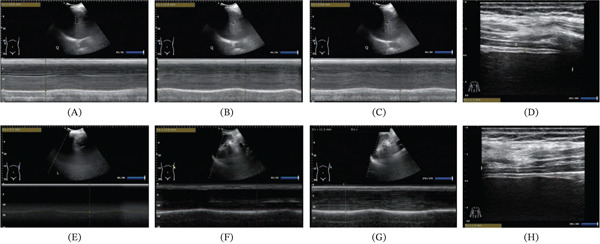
The ultrasound image shows the changes in bilateral diaphragm activity and diaphragm thickness.
